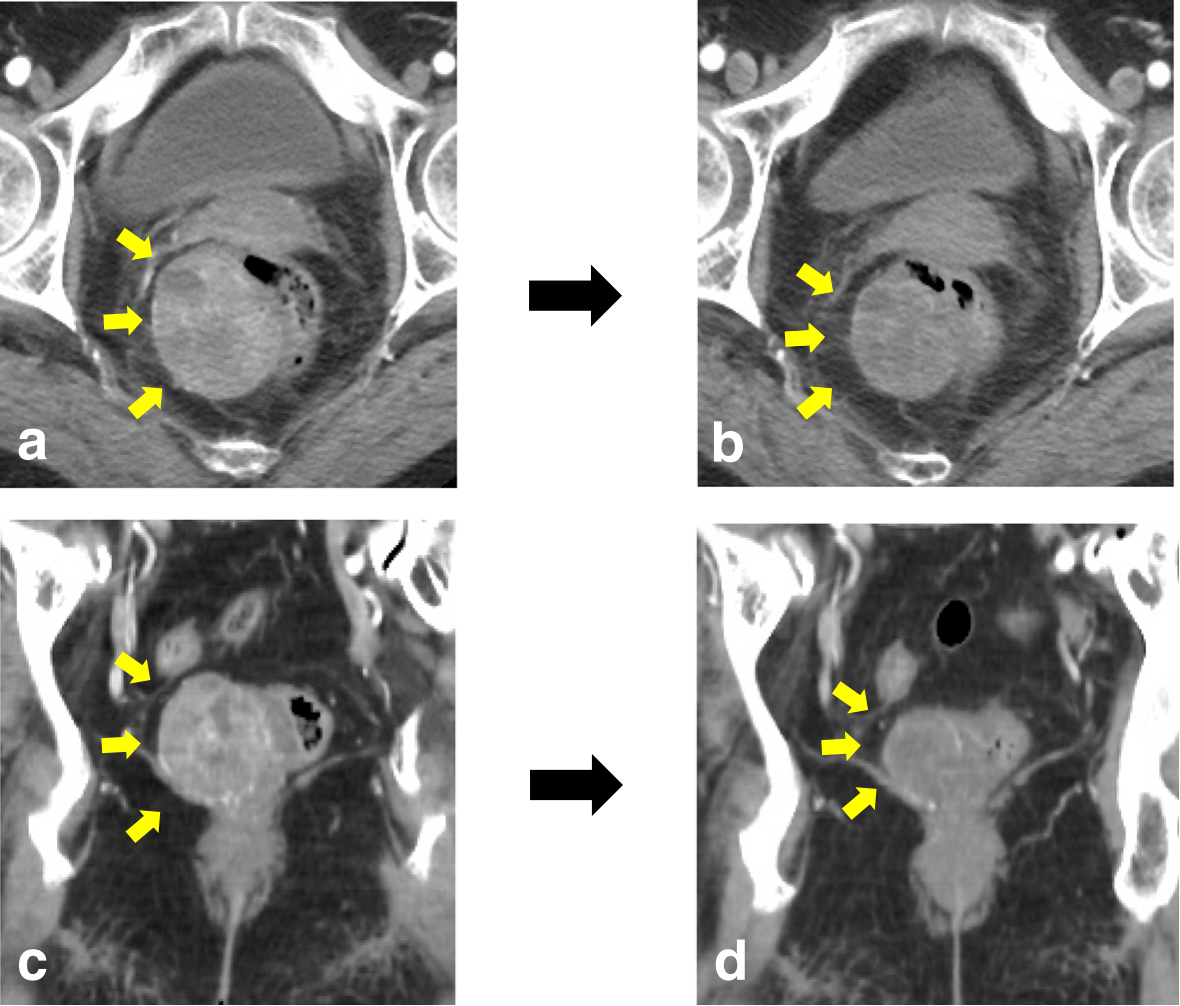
a Coronal CT images showed an enhanced tumor measuring 5.3 × 4.2 cm behind the rectum. b After the treatment of imatinib, coronal CT images revealed that the tumor size decreased to 3.5 cm. c Axial CT images showed an enhanced tumor at the right posterior side of the rectum. d After treatment of imatinib, axial CT images revealed that the tumor size decreased to 3.5 cm
